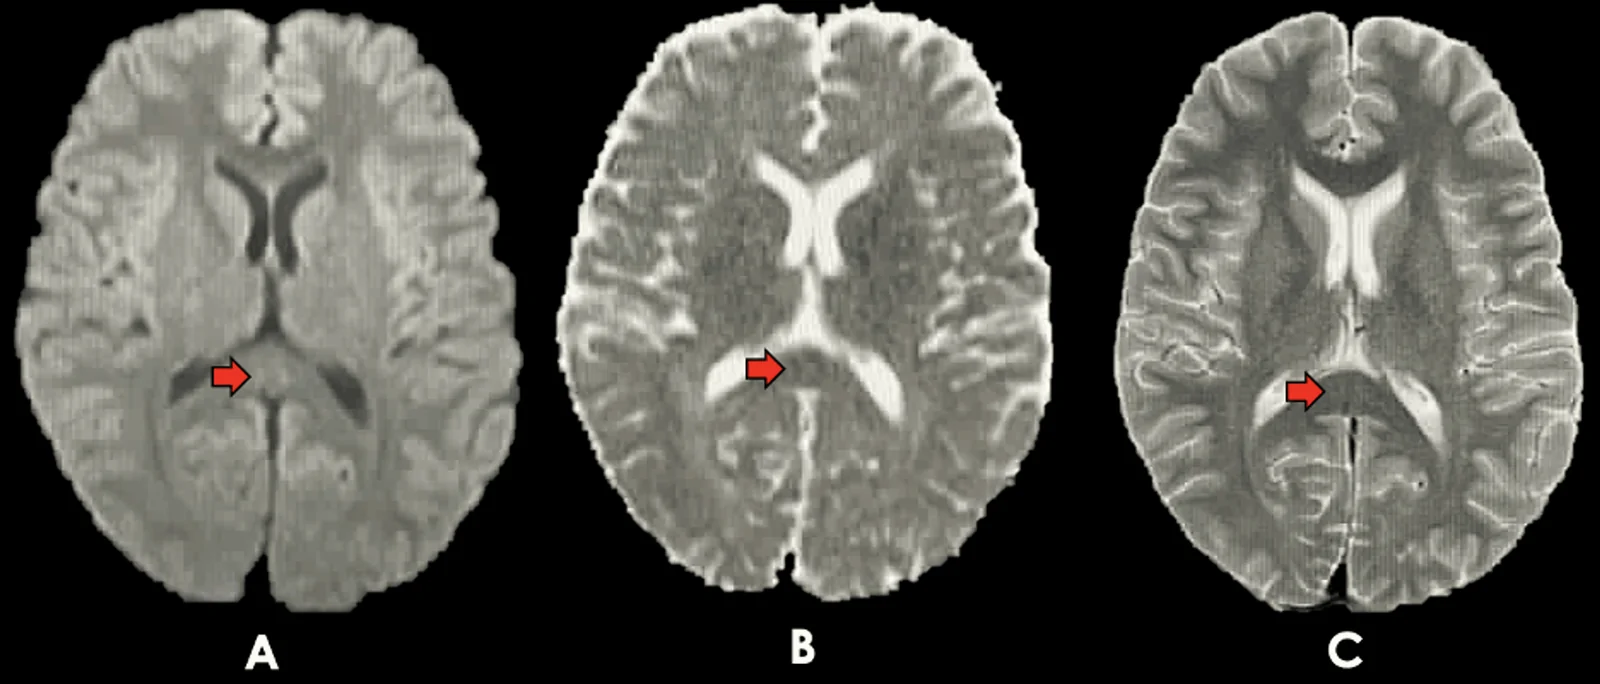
(A) Axial diffusion-weighted imaging (DWI) demonstrates complete resolution of the previously noted diffusion restriction in the splenium of the corpus callosum, with (B) normalization of signal on the apparent diffusion coefficient (ADC) map. (C) Axial T2-weighted sequences show resolution of the previously observed splenial hyperintensity. No new parenchymal signal abnormalities, hemorrhage, or abnormal enhancement are identified. MRI = magnetic resonance imaging
